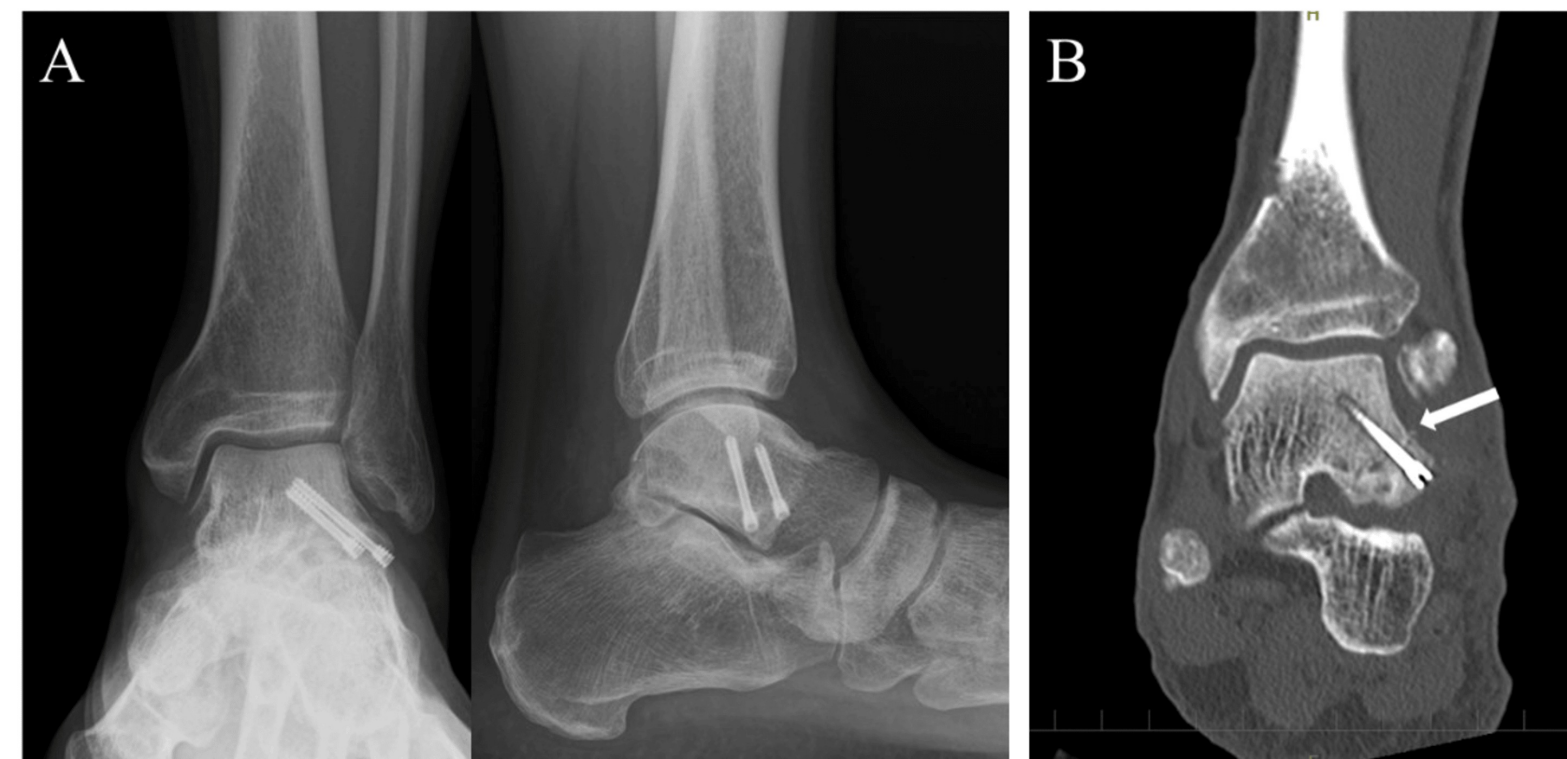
Plain radiographs and CT findings. A: Plain radiographs at one year after surgery. B: CT showed bone union.
